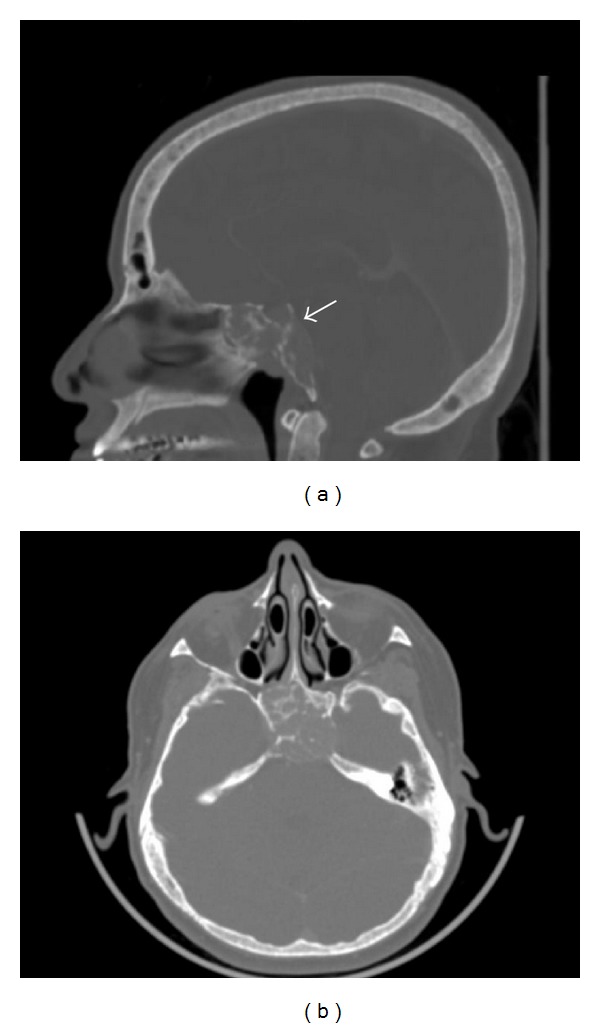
Computed tomography of skull that shows a heterogeneous, osteolytic lesion that involves sphenoid corpus and clivus. (a) Sagittal section. (b) Axial section.
